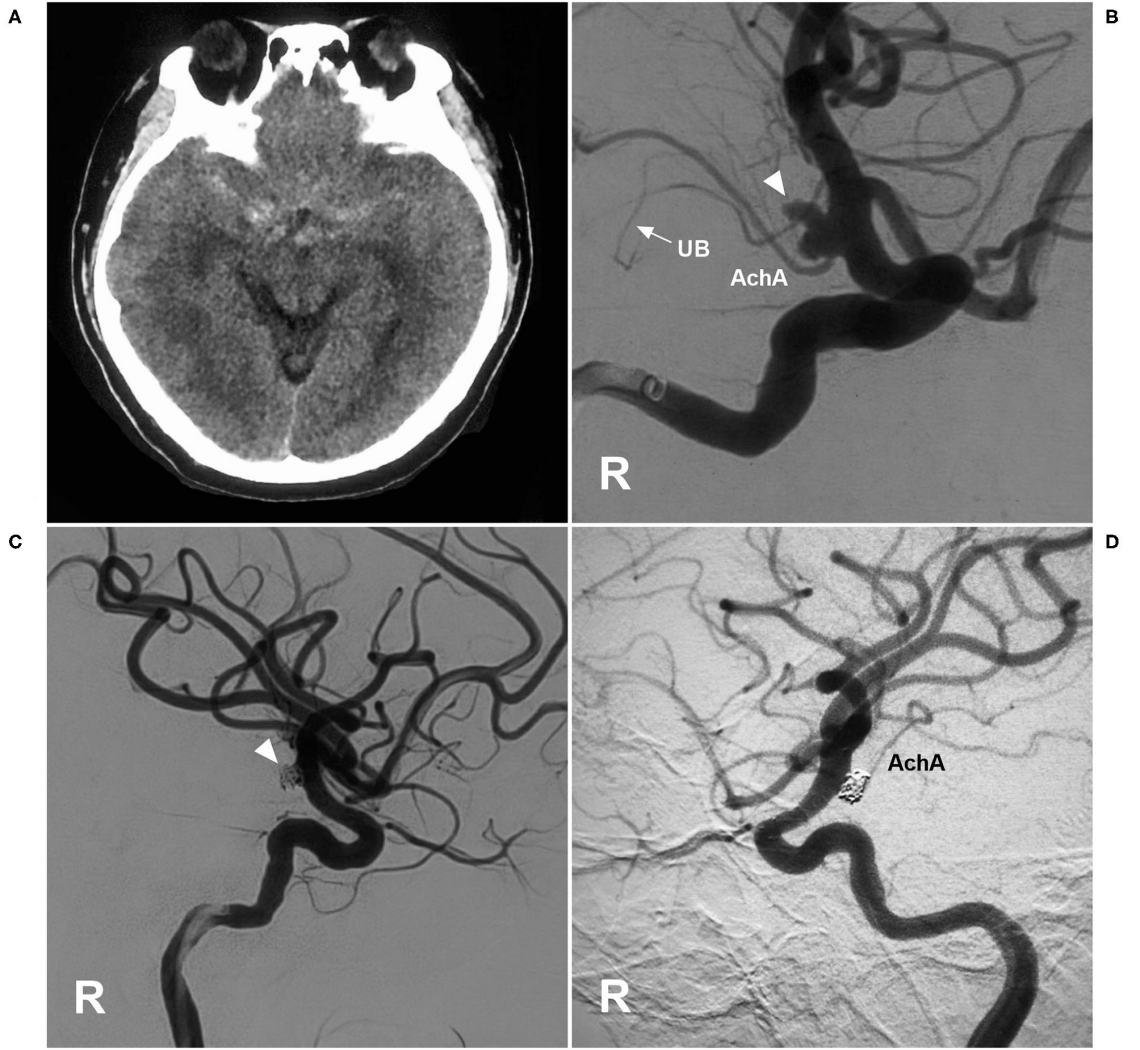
EVT of an AchA aneurysm with AchA occlusion. (A) CT showing subarachnoid hemorrhage. (B) DSA of the right ICA showing a type II AchA aneurysm (triangle). The AchA was hyperplastic, and the uncal branch (UB, arrow) could be seen. (C) DSA of the right ICA showing that the aneurysm was coiled (triangle); however, the AchA was occluded, without new neurological deficits. (D) Follow-up DSA showing the aneurysm with embolization with MRCC grade I, and the AchA reappeared. AchA, anterior choroidal artery; CT, computed tomography; DSA, digital subtraction angiography; EVT, endovascular treatment; ICA, internal carotid artery; MRCC, Modified Raymond-Roy Classification; R, right; UB, uncal branch.
